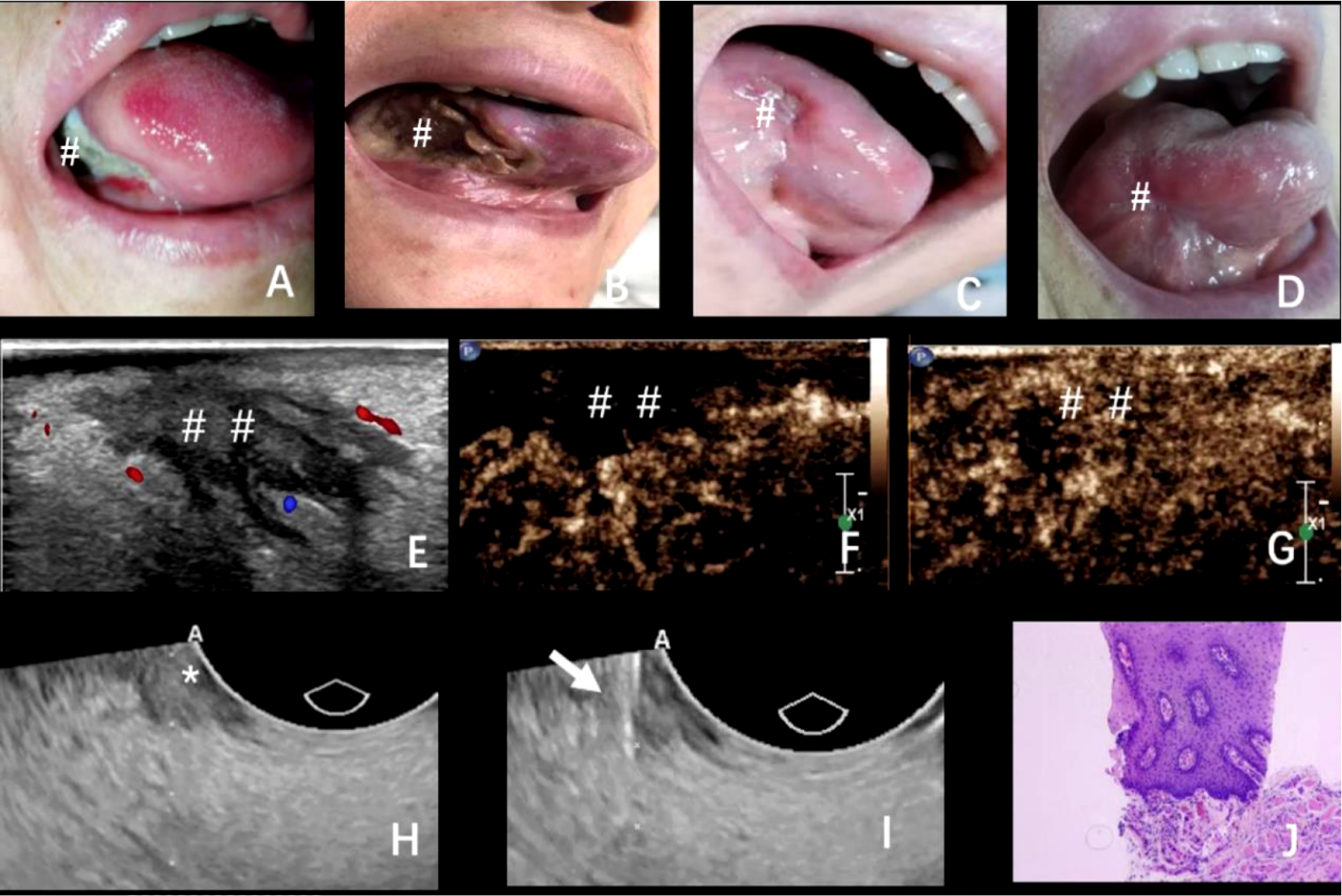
(A–D) The changes in the ablation area after the operation at 4, 7, and 21 days and 2 months; (E–G) 2 months after the operation, ultrasound examination showed that the ablation area showed patchy low echo, clear boundary, irregular shape, and punctate blood flow signal. Contrast-enhanced ultrasound showed that the ablation area showed slow and low enhancement in the arterial phase and equal enhancement in the venous phase; (H, I) Puncture biopsy of different parts of hypoechoic area under the guidance of intracavitary ultrasound; (J) Pathological biopsy showed that no cancer cells were found in the ablation area. The arrow: Puncture biopsy needle; * and ^#^The exact location of the lesions; ^##^The area of ablation.
